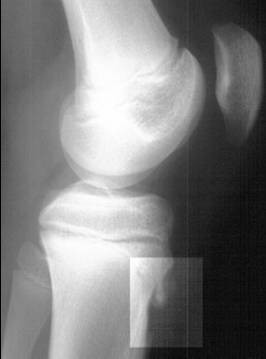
Osgood-Schlatter disease. Lateral radiograph of the knee demonstrating fragmentation of the tibial tubercle with overlying soft tissue swelling. (Radiograph courtesy of BC Children's Hospital)
